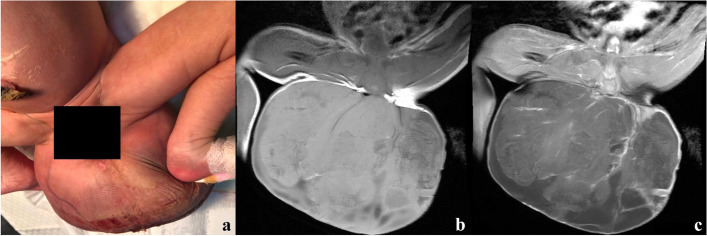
a Large sacrococcygeal mass causing abduction of both legs. b Unenhanced MRI of the pelvis in coronal T1W view shows large exophytic sacrococcygeal mass with heterogenous signal intensity. c Post-gadolinium MRI of the pelvis in coronal T1W view shows minimal enhancement of the exophytic mass
